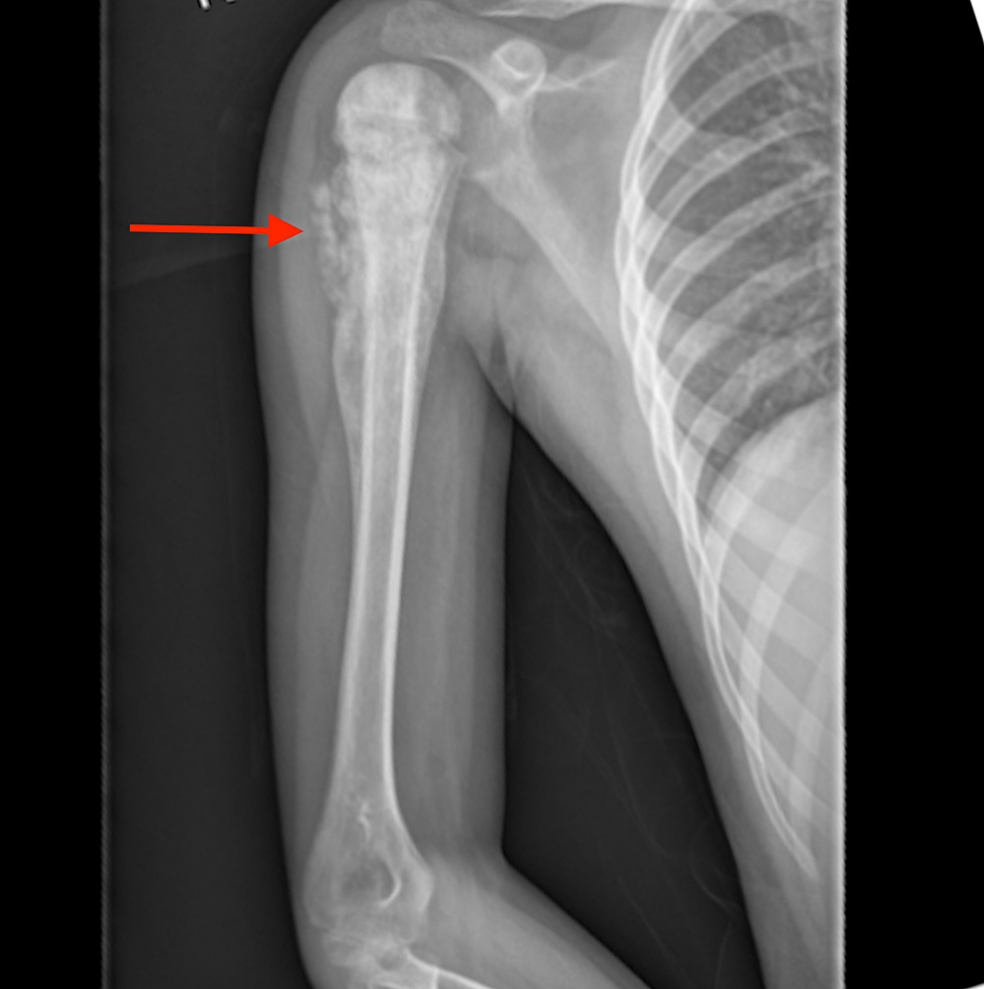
Anterior-Posterior (AP) radiograph depicts the classic periosteal elevation resulting in the Codman triangle. It also highlights the periosteal reaction resulting in cloud-like bone formation. The tumor seen involves the metaphyseal region of the humerus with extension into the diaphysis. There is also a wide zone of transition which has been shown to be a feature of aggressive lesions.
